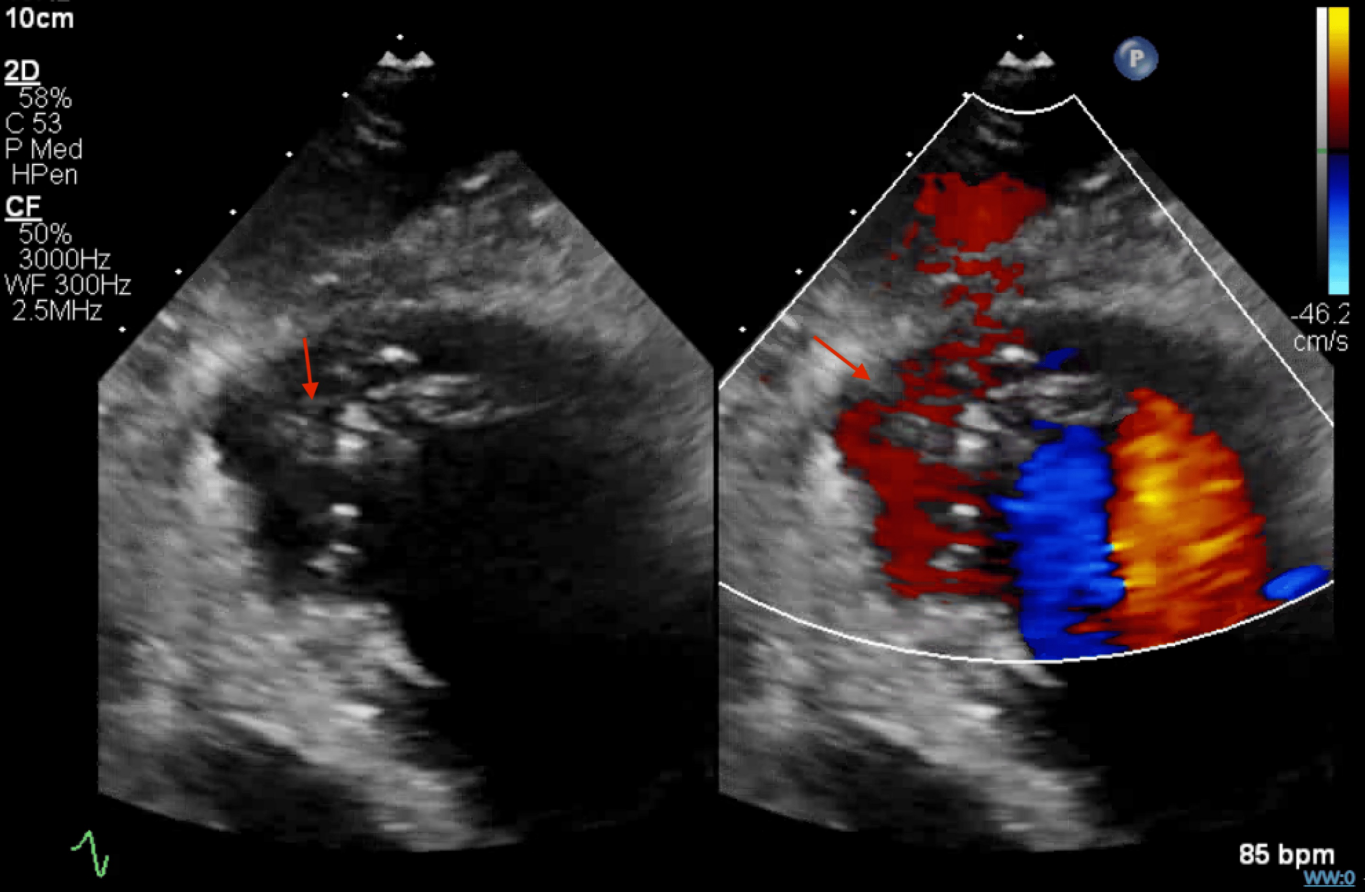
Color Doppler imaging in systole shows flow through intertrabecular recesses The red arrows show some of the trabeculations in the left ventricle.
